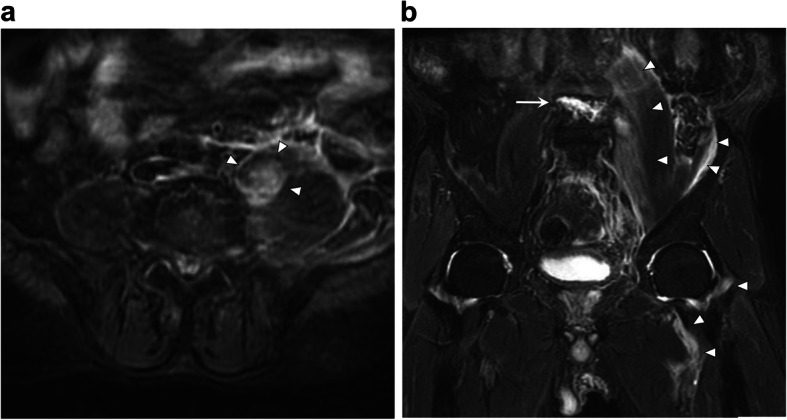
Fast STIR magnetic resonance imaging of iliopsoas hematoma. a Axial image shows hyperintense areas corresponding to hematoma into the psoas major muscle (arrowhead). b Coronal image shows the fractured L4 vertebra (arrow) and reveals that the hematoma expanded to not only the psoas muscle but also the iliac muscle and around the hip joint (arrowhead)
